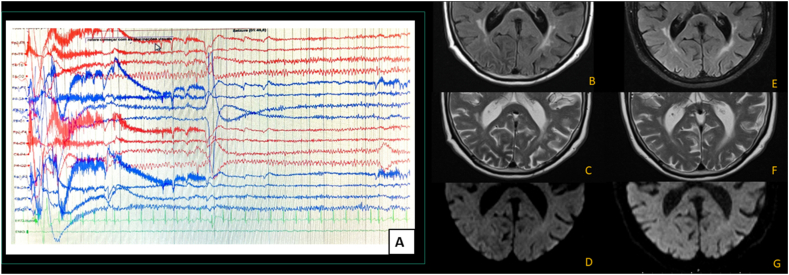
A. Ictal electroencephalogram showing occipital focal seizures following left oculo-cephalic version. There is associated right occipital diffuse cortical dysfunction. Initial brain magnetic resonance imaging (B,C,D) showing decreased signal intensity in Fluid-attenuated inversion recovery (B) and T2 (C) with increased cortical sign (D) on diffusion weighed imaging in the right middle occipital region. Follow up brain imaging (E,F,G) showing resolution of the brain signal abnormality.
